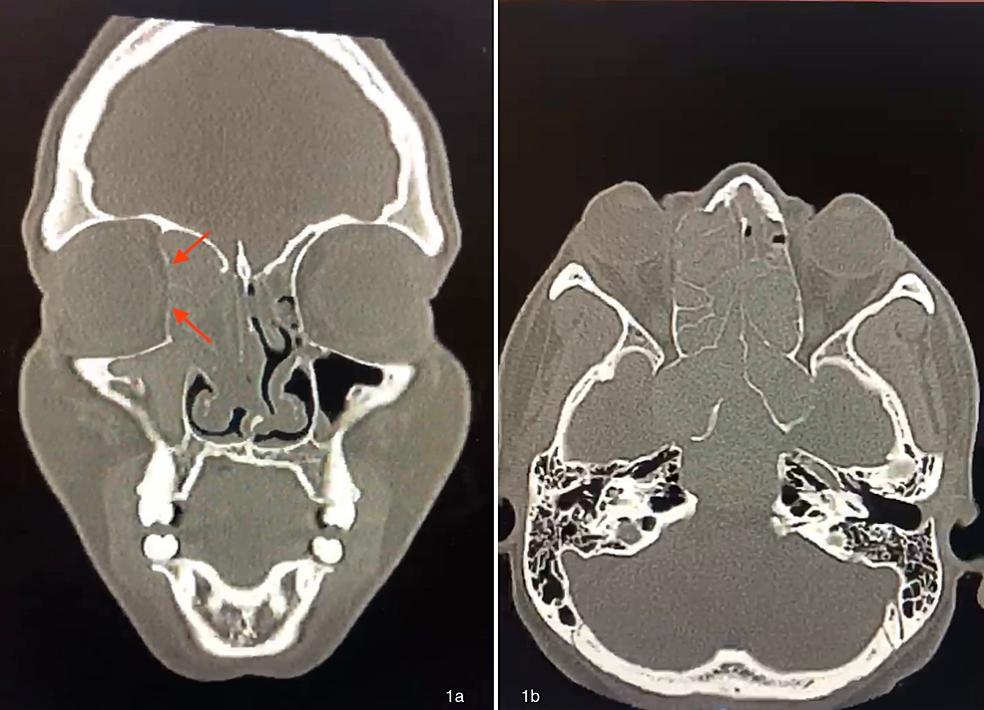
1a: Non-contrast coronal CT scan showing a bilateral pan sinus process with expansion causing thinning of the right medial orbital wall (arrows). 1b: Non-contrast axial CT scan showing bilateral ethmoid and sphenoid sinus process with posterior expansion with mass effect on the anterior cranial fossa and cavernous sinus and displacement of the pituitary gland
